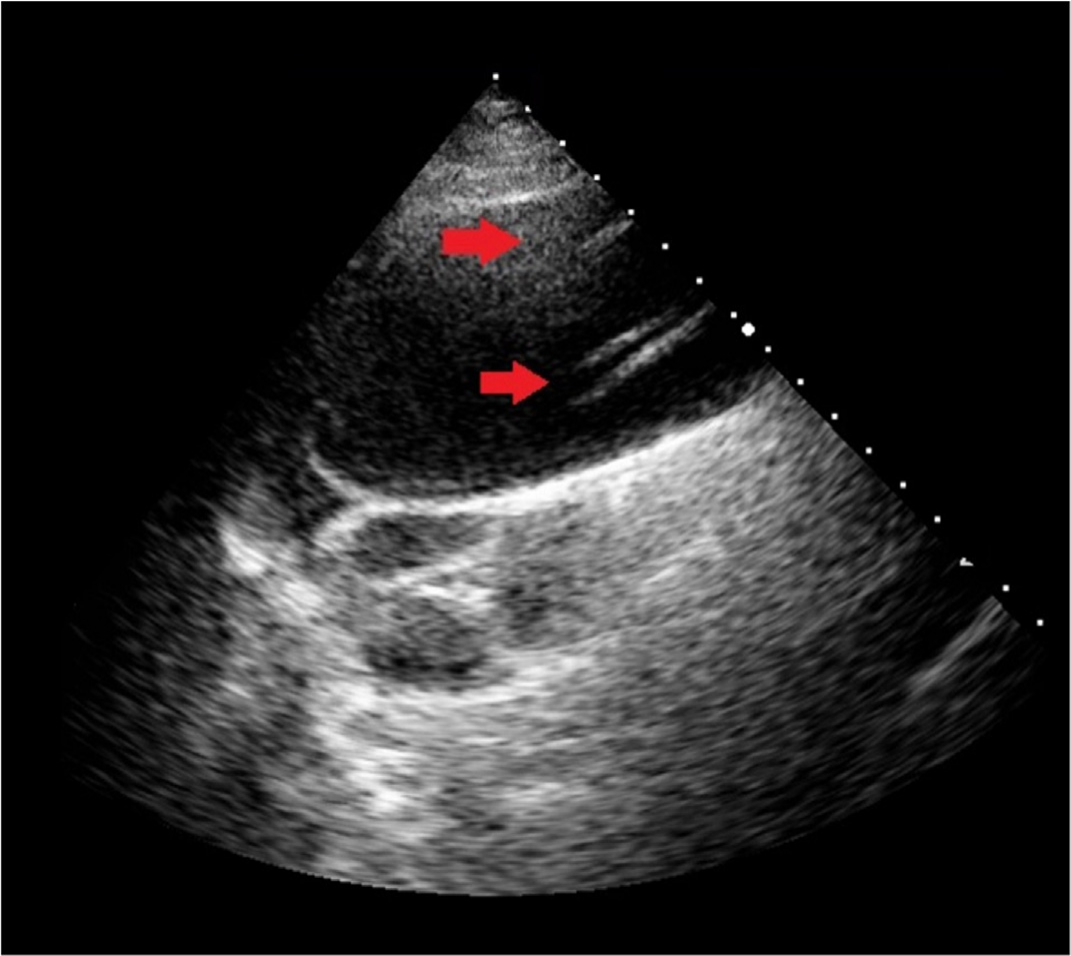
Aortic Dissection The right parasternal view definitively highlighted the intimal flap (red arrows).
